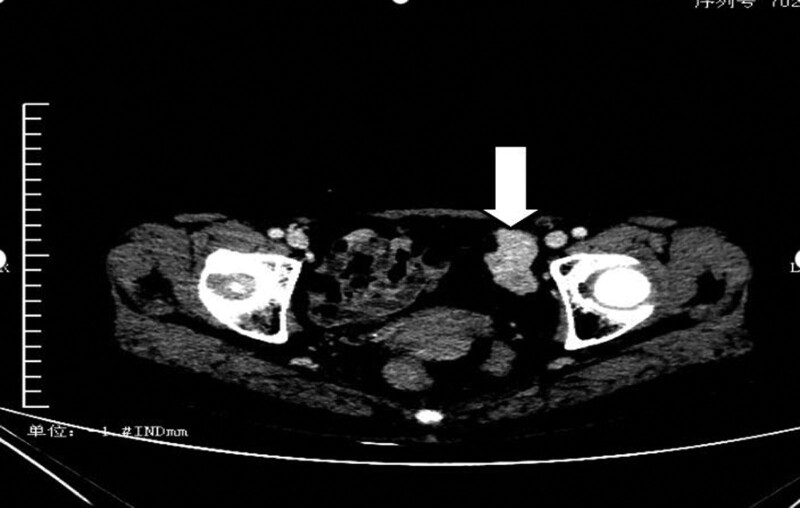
The CT scan indicated a long soft tissue mass near the left iliac blood vessel, which was interpreted as a benign lesion. CT = computed tomography.
